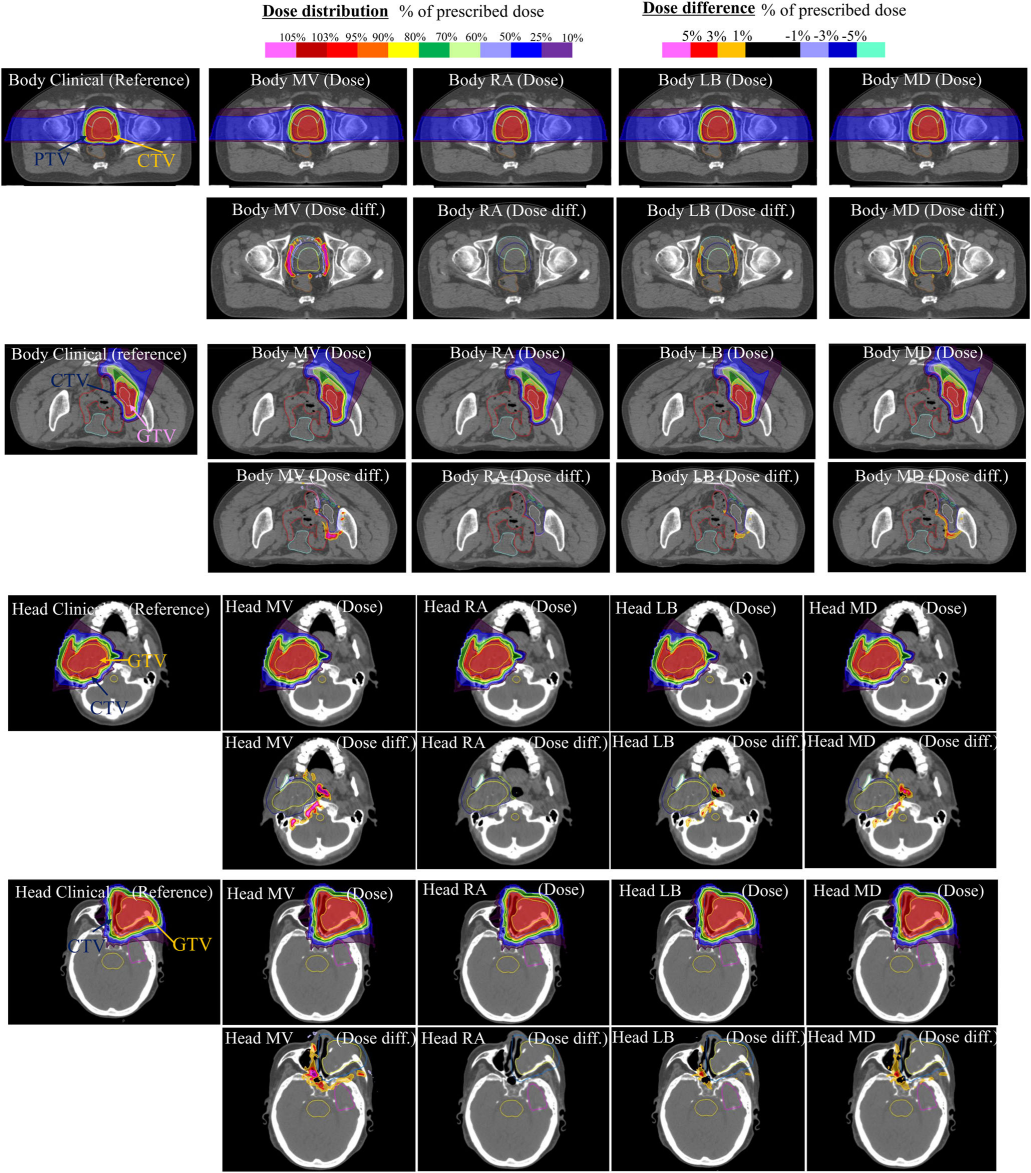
Dose distributions of the clinical treatment planning reference dose and dose calculated by CT with each four CT scan protocol CT‐SPR conversion table and their difference distributions in prostate and postoperative pelvic recurrence of colorectal cancer cases. CT, computed tomography; SPR, stopping power ratio.
